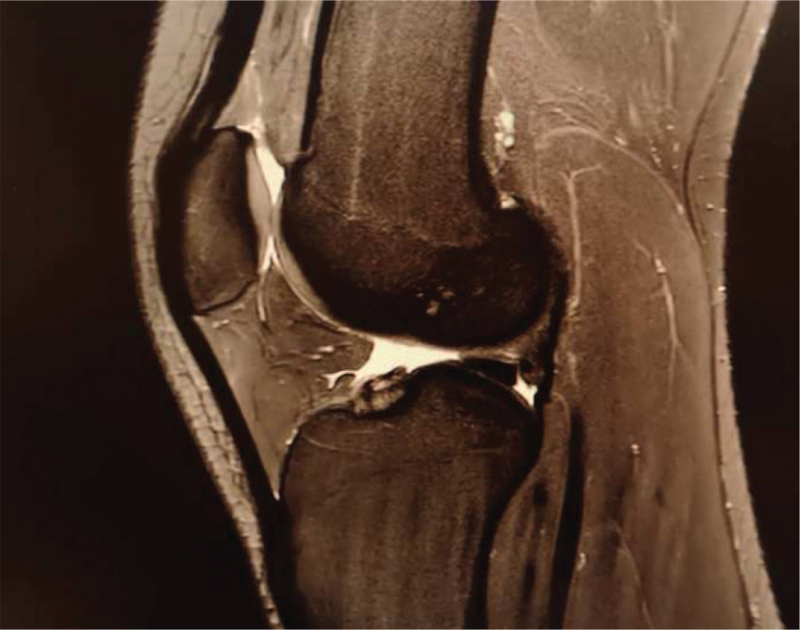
MRI image of the 19-yr-old female karateka; no pathological changes of the tibial tuberosity and ligaments are observed.
